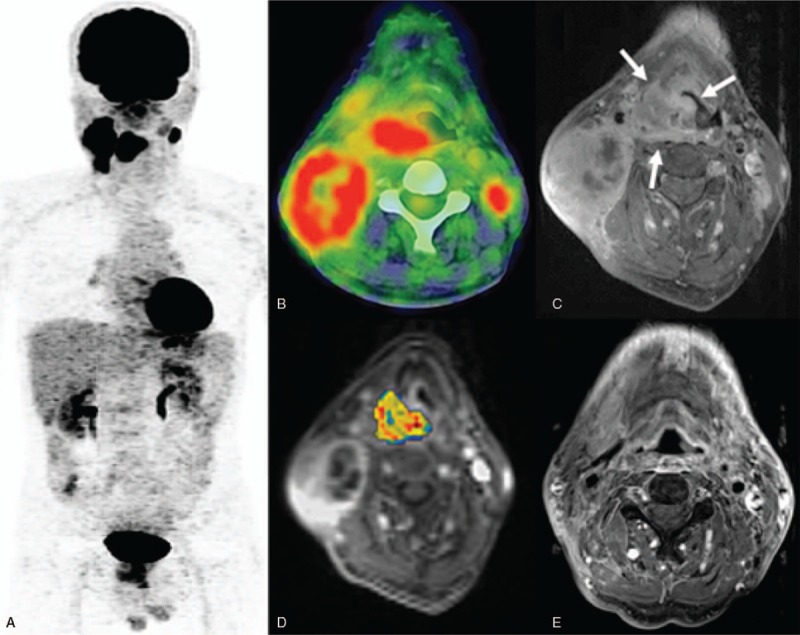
1. (a) A PET image of a 40-year-old male patient with stage IVa hypopharyngeal squamous cell carcinoma and a score of 4. (b) The ^18^F-FDG PET/CT image showed a high primary tumor entropy value (7.217). (c) A left hypopharyngeal tumor was also identified on the corresponding axial-enhanced T1-weighted MRI image. (d) A DCE-MRI image with an overlaid volume transfer rate constant (K^*trans*^) map of the primary tumor exhibited a low value of 0.317 min^−1^. (e) The patient had a persistent tumor at the locoregional site (arrow) and died 9 months thereafter. 4–2. (a) A positron emission tomography (PET) image of a 58-year-old male patient with stage IVb hypopharyngeal squamous cell carcinoma staging and a score of 1. (b) The ^18^F-FDG PET/CT image showed a low entropy value (6.785). (c) The corresponding axial-enhanced T1-weighted MRI also revealed a bulky right hypopharyngeal tumor. (d) The corresponding DCE-MRI with an overlaid volume transfer rate constant (K^*trans*^) map had a high value (0.655 min^−1^). (e) A post-treatment MRI showed complete remission of the primary tumor. After 60 months of follow-up, the patient is still alive without recurrences. CT = computed tomography, DEC = dynamic contrast-enhanced, MRI = magnetic resonance imaging, PET = positron emission tomography.
